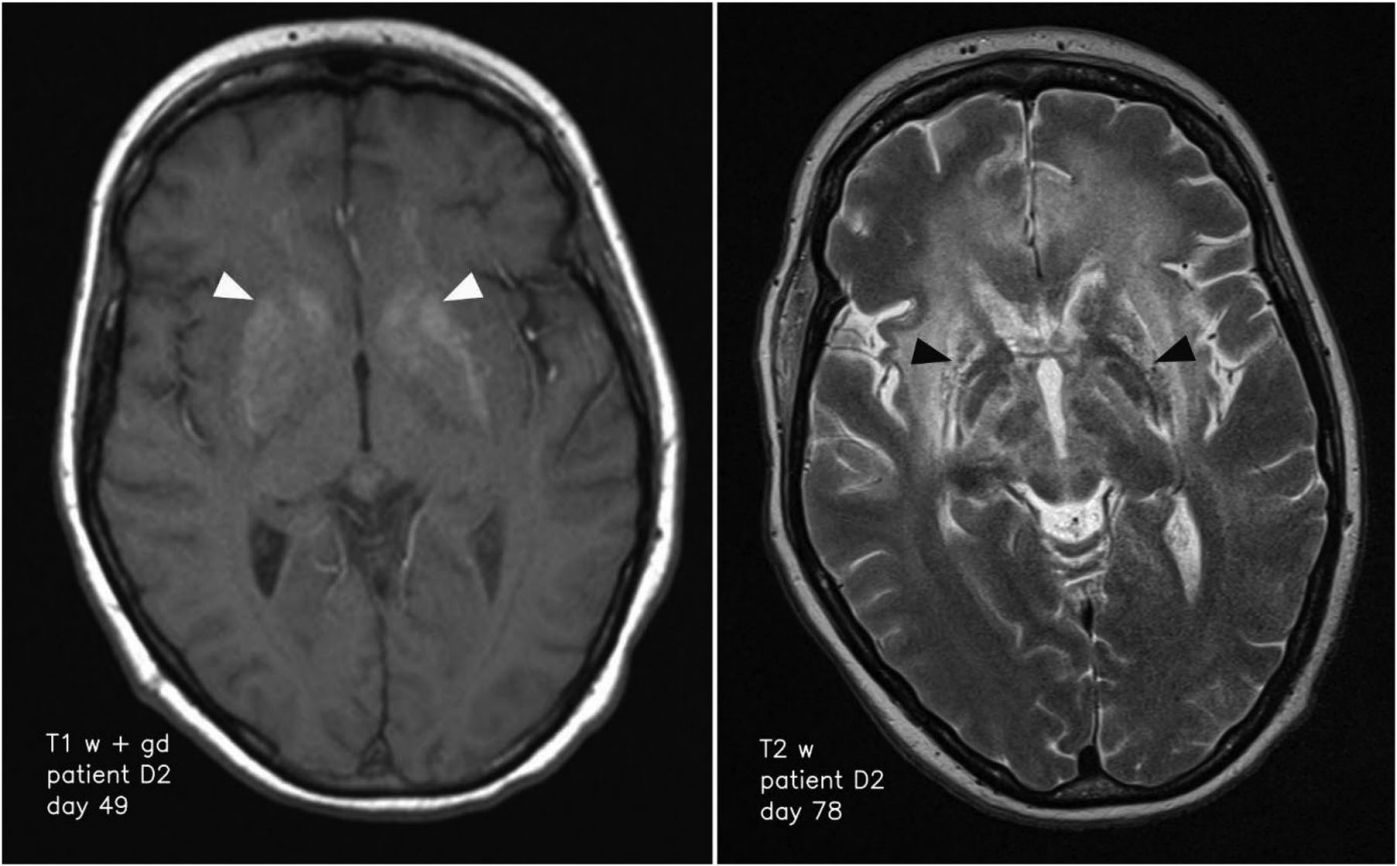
Transformation of the basal ganglia and respective changes on MR imaging over time. High signal of basal ganglia on T1 weighted imaging on day 49 after symptom onset (left; white arrowheads) and structural damage to the basal ganglia 72 days after symptom onset (right; black arrowheads).
